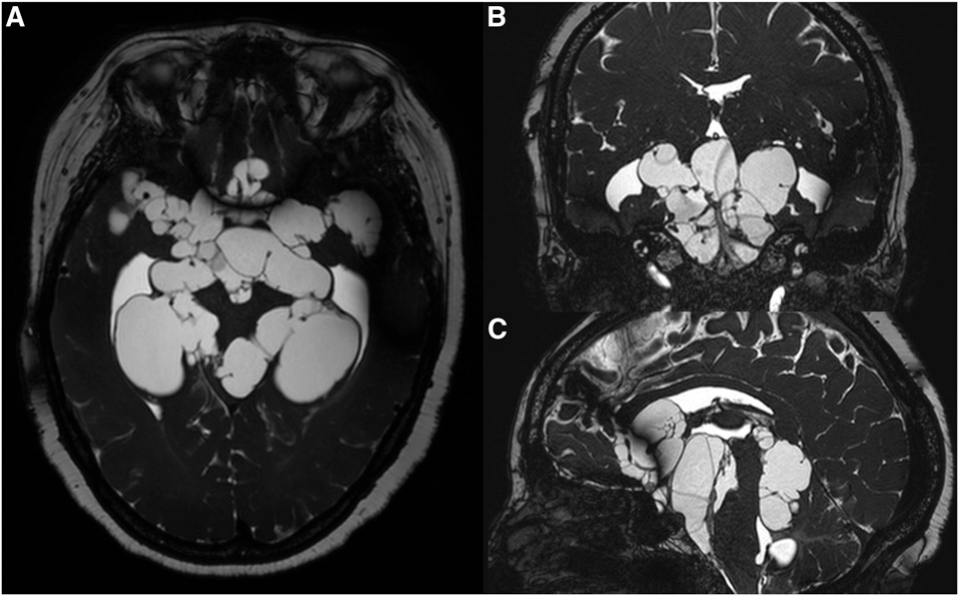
Magnetic resonance imaging of the head with gadolinium contrast in fast imaging employing steady-state acquisition sequence showing extent of cystic lesions in the basal cisterns in (A) axial, (B) coronal, and (C) sagittal sections.
